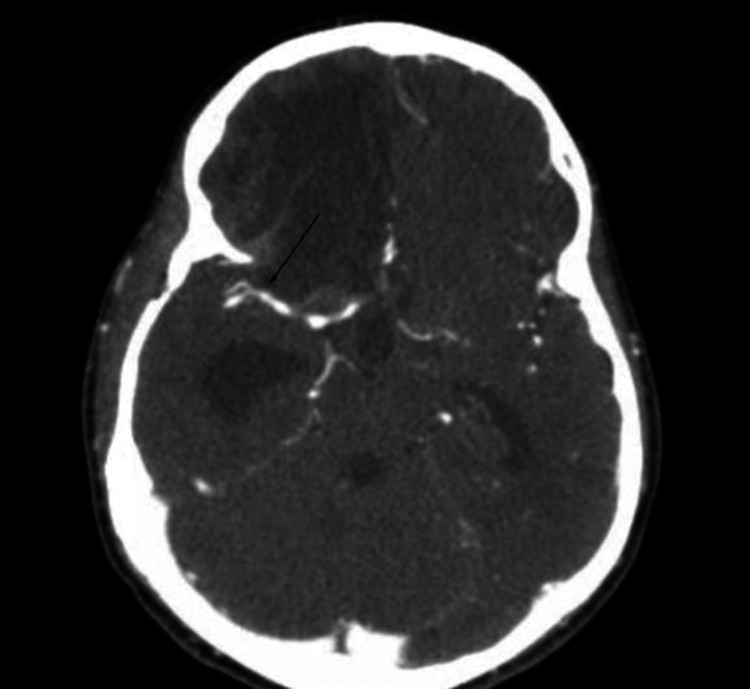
Follow-up brain CT angiography axial image performed two months after admission shows no evidence of the pseudoaneurysm (arrow indicates the location of the previously identified pseudoaneurysm) with frontal encephalomalacia and asymptomatic ex-vacuo ventriculomegaly
